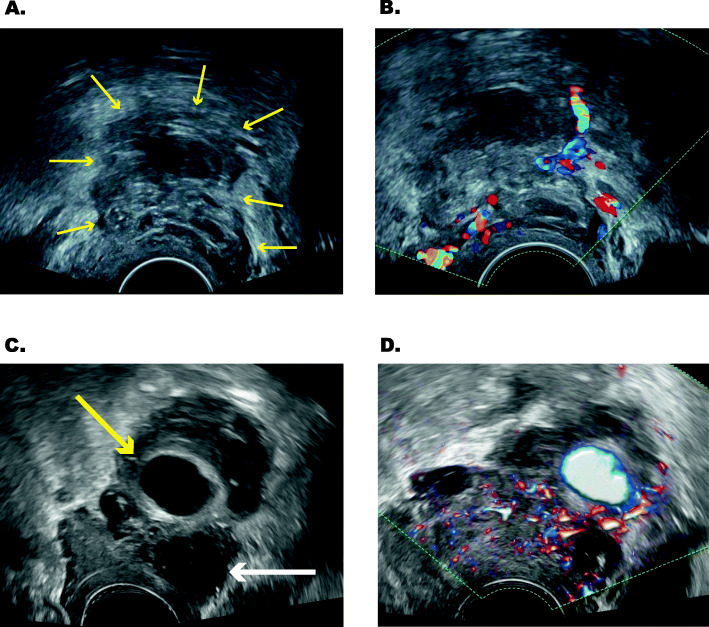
Development of the UPA over 2 weeks by ultrasound. Visit 1 with a. Ultrasound image of the ill-defined solid mass in the adnex at the initial presentation at 23 + 2 gestational weeks. The arrows mark the outer margins of the lesion. An endometrioma is not clearly visible. b. Corresponding Doppler image of the left adnexa showing moderate blood flow (Color Score 3) in the lesion. Visit 2 with c Ultrasound Image of the same lesion on the follow up at 25 + 2 gestational weeks. On the lower right there is an unilocular mass suggestive of endometrioma. In the center there is a pulsating vessel (UPA) of about 2 cm with most likely haematoma surrounding the UPA and d. corresponding Doppler image confirming blood flow in the vessel.
